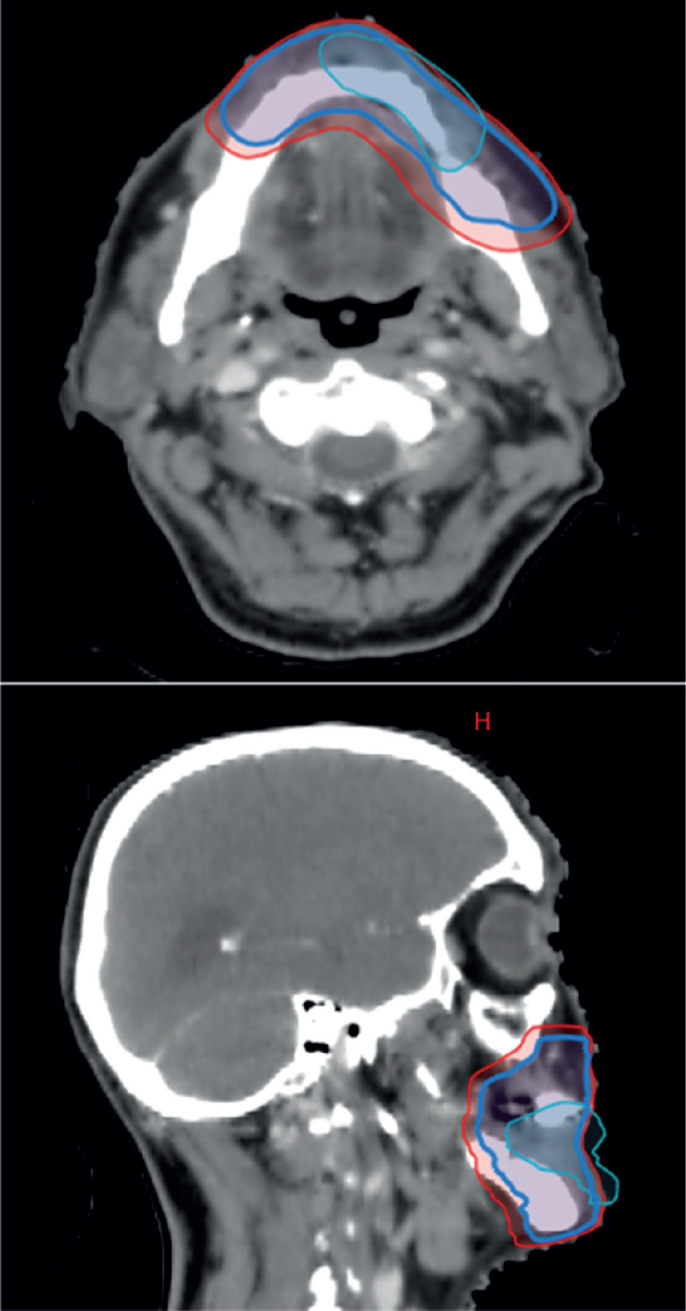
CT-based RT planning, representing pre-chemotherapy GTV, which has now regressed in cyan, CTV and PTV in red.
